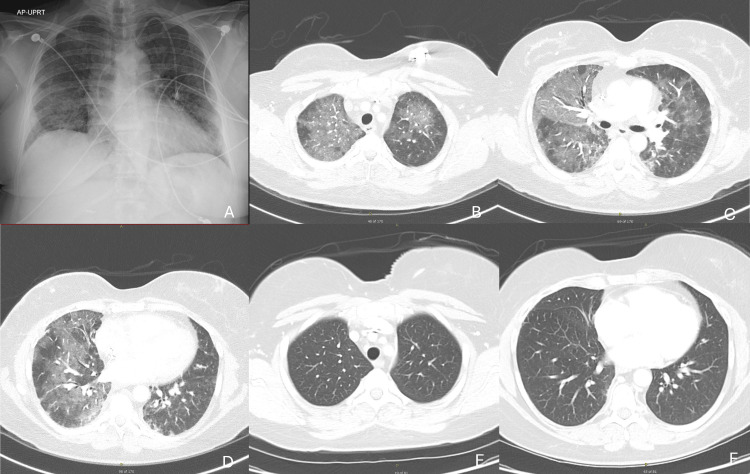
Chest radiograph (A) and CT of chest (B-D) on admission and follow-up CT of chest showing resolution of ground-glass opacities (E, F)
